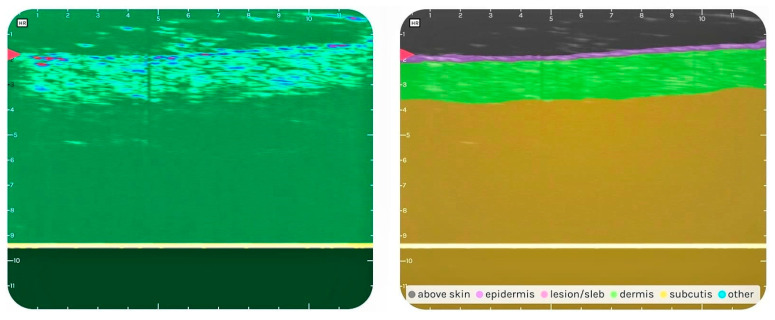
Ultrasound images of the lesion: Ultrasound image (left) and ultrasound image analyzed with SkinAid App (right). The ultrasound image reveals an absence of pathological alterations in the affected area. The appearance of the dermis, the subepidermal region, is without abnormal modifications except for a slight increase in the thickness of the dermis. Altered echogenicity cannot be observed.
